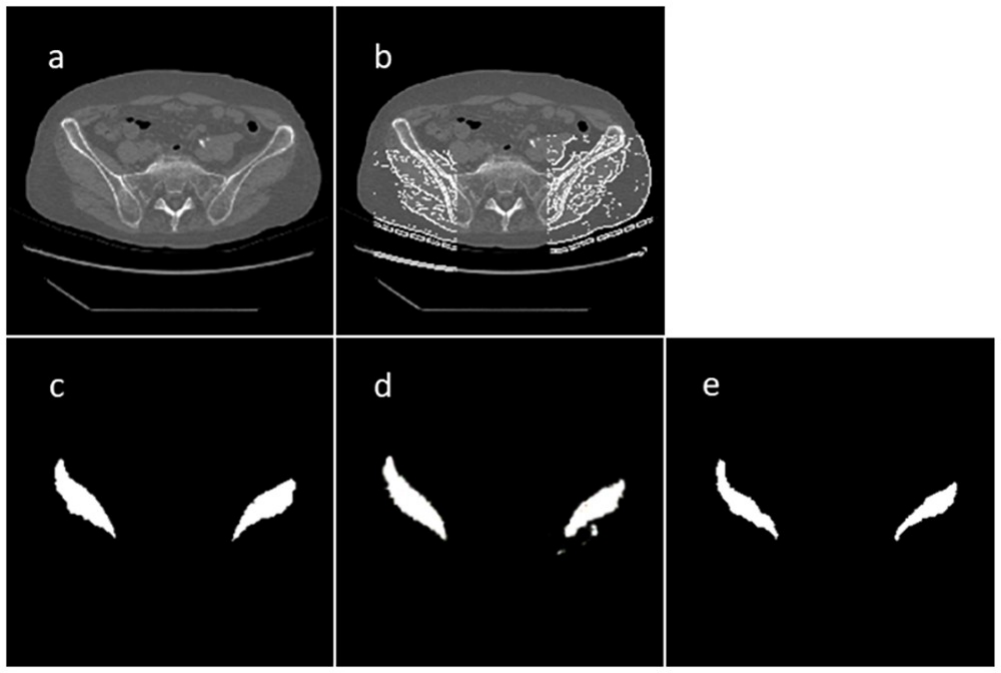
CT and segmentation images: (a) original CT image, (b) guide image using Canny edge detector, (c) manual segmentation image of GMd contours, (d) Auto-segmentation image obtained using learning model with beta1 = 0.9, L1_weight = 1000, and 100 epochs, and (e) manually segmented image prepared by another operator.
